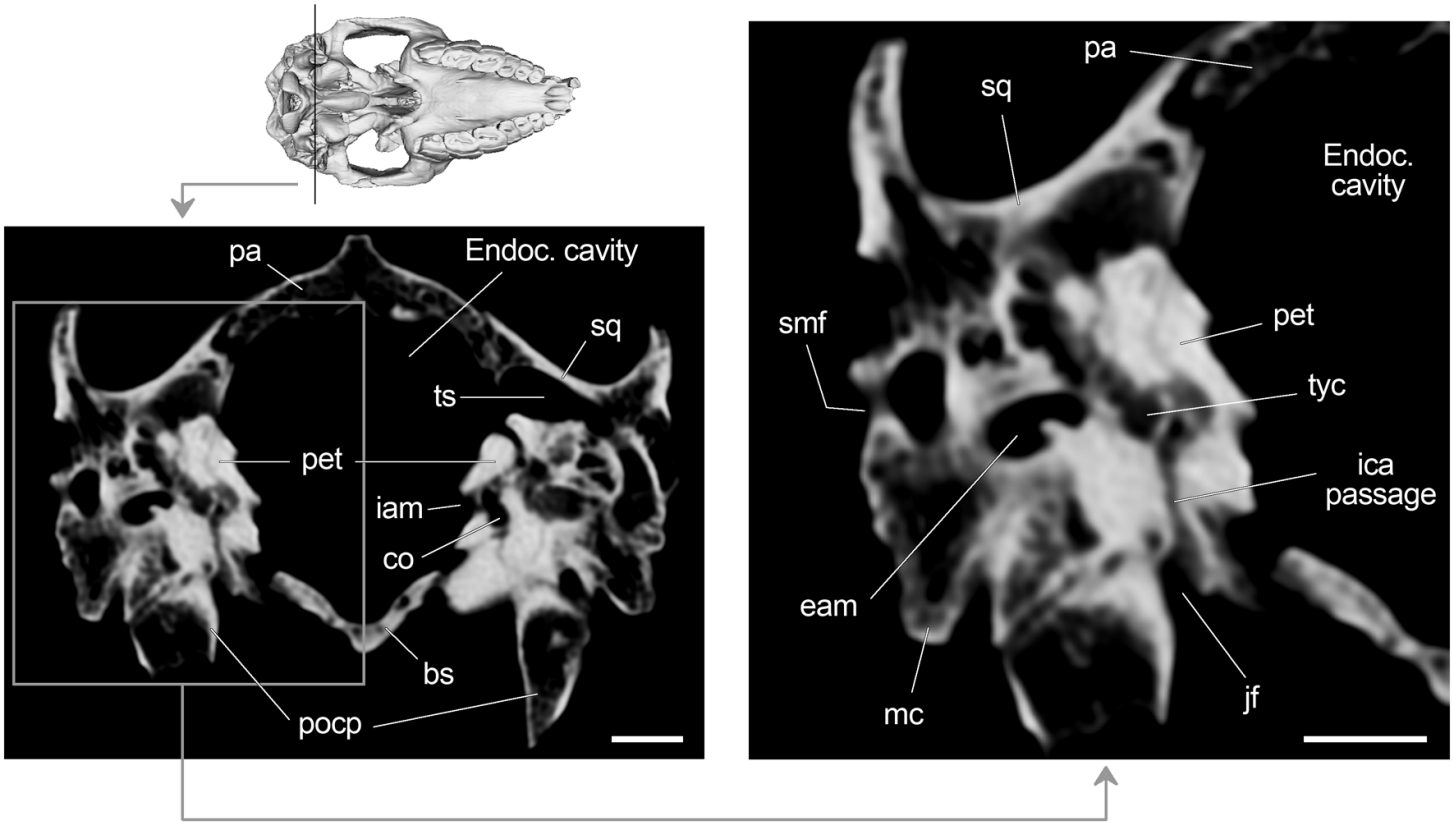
Axial CT slice of *R*. *equinus* (MPEF PV 695) at level of the jugular foramen. Anatomical abbreviations: bs, basisphenoid; eam, external auditory meatus; ica, internal carotid artery; jf, jugular foramen; mc, meatal crest; pa, parietal; pet, petrosal; pocp, paraoccipital process; smf, suprameatal foramen; sq, squamosal; ts, temporal sinus; tyc, tympanic cavity. Scale bar equals 1 cm.
